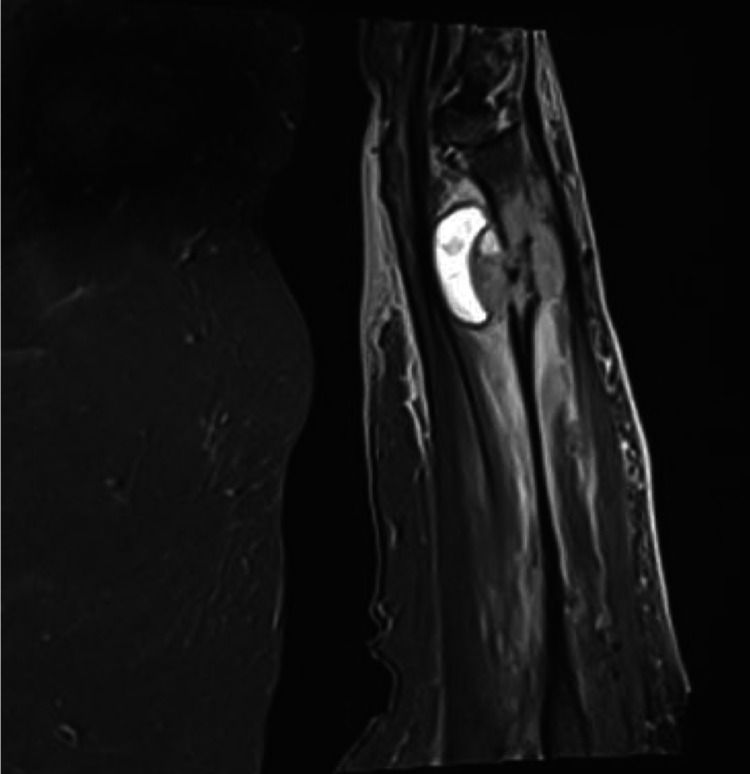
MRI of right arm MRI scan of the right distal radius, showing the metastatic lesion causing bone destruction.
